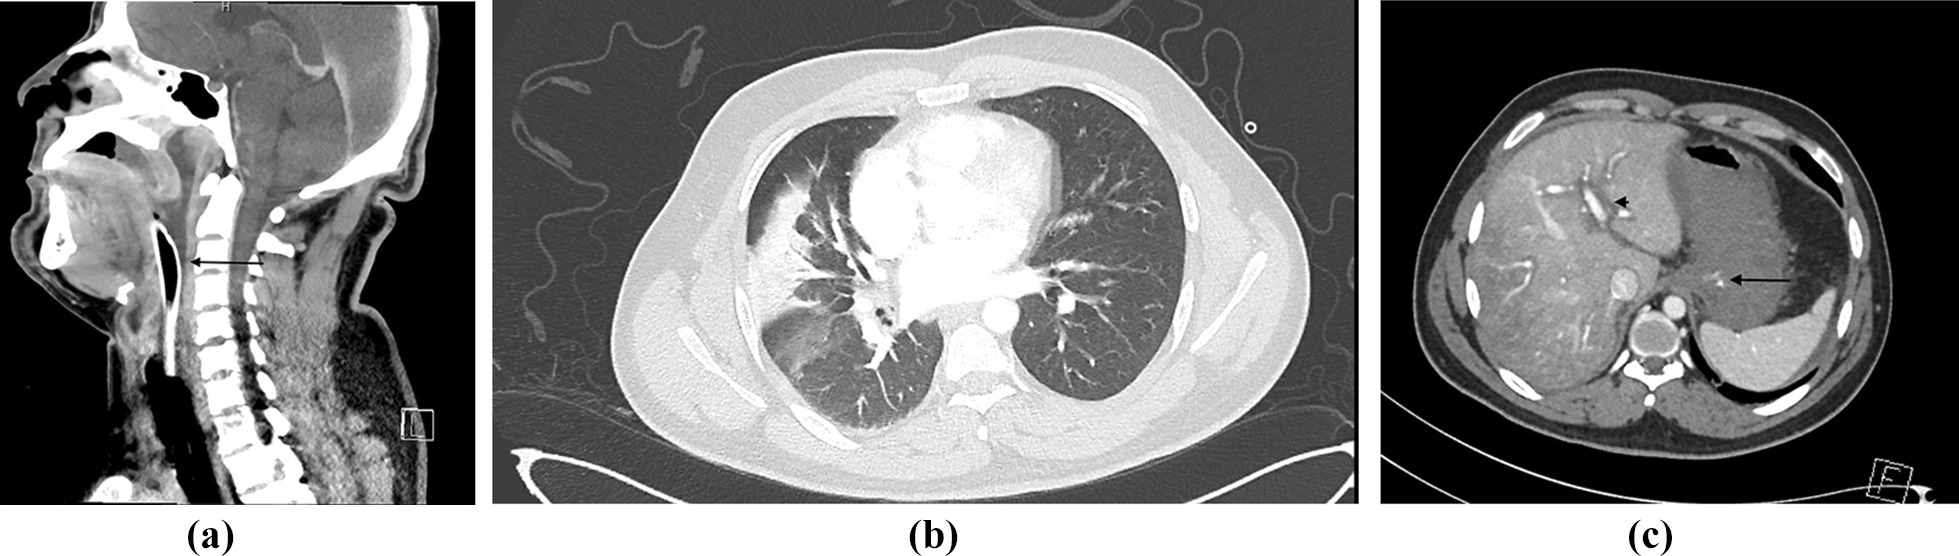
a Computed tomography of the neck with an arrow showing retropharyngeal swelling. b Computed tomography of chest showing aspiration pneumonitis in the right lung. c Computed tomography of the abdomen with an arrowhead showing periportal swelling and arrow showing the stomach filled with blood with associated contrast blush
